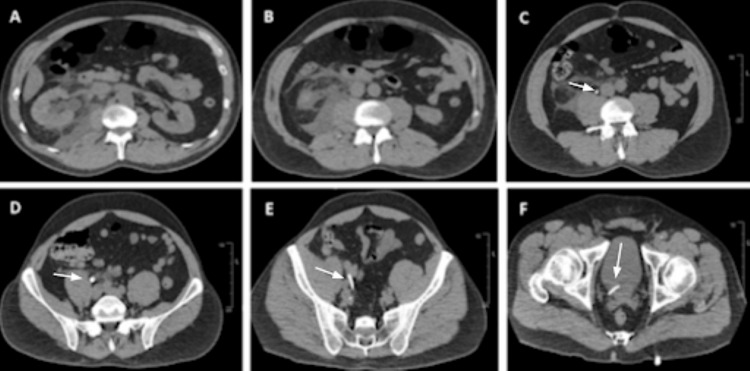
Noncontrast enhanced CT imaging of the abdomen and pelvis demonstrating right hydroureteronephrosis (A,B) with a linear high-density structure in the distal ureter extending into the bladder concerning for ureteral stent (C-F)
